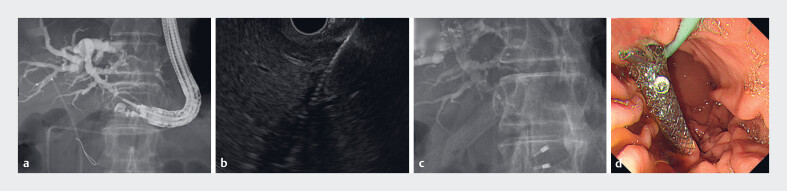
Images during biliary drainage using endoscopic ultrasound-guided hepaticogastrostomy
